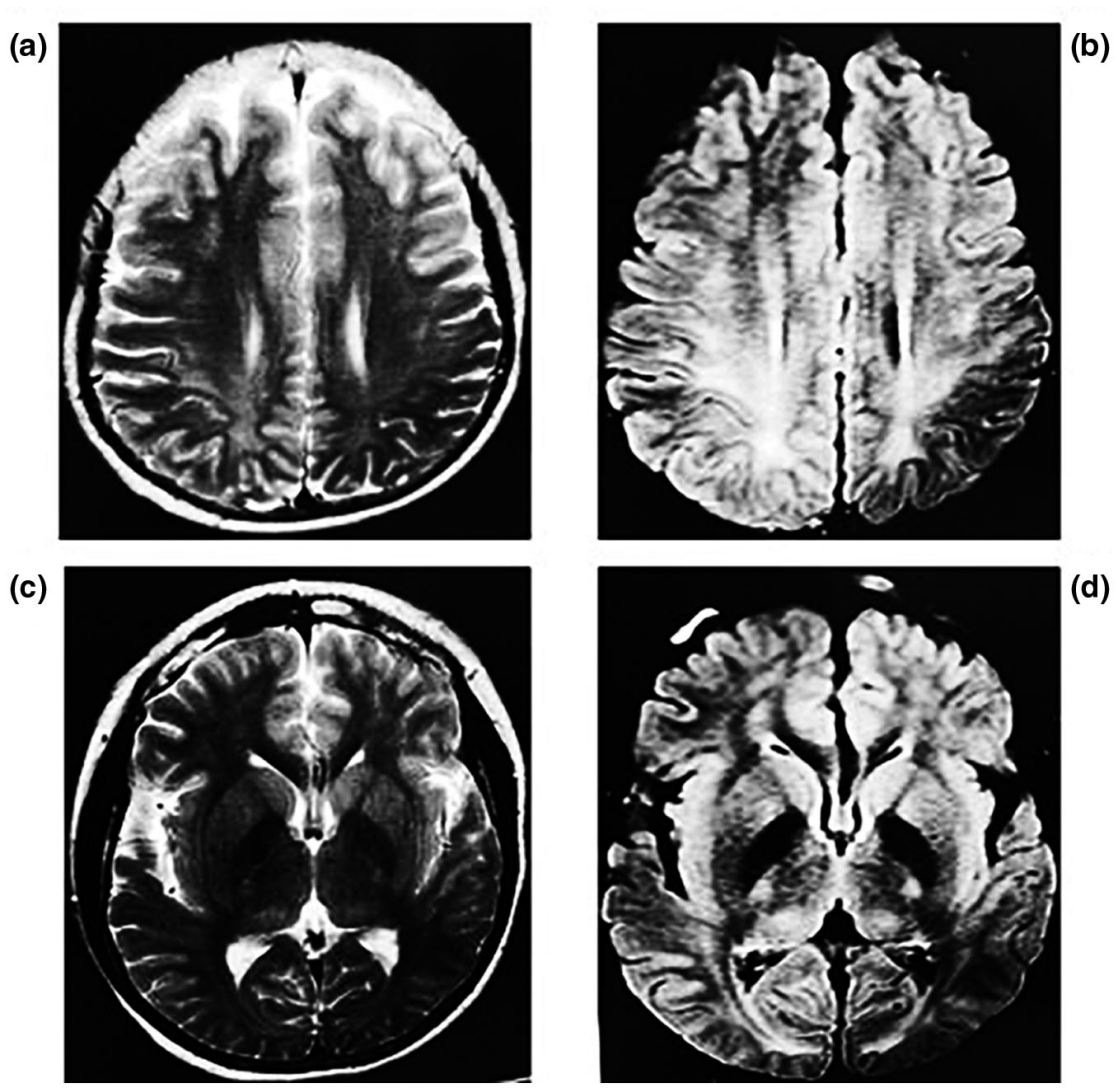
Magnetic resonance imaging (MRI) of the brain. (a and b) T2‐weighted and T2 FLAIR images present bilateral symmetrical patchy hyperintensity in the white matter of the parietal lobe. (c and d) T2‐weighted and T2 FLAIR images show symmetrical flaky hyperintensity in the bilateral thalamus
